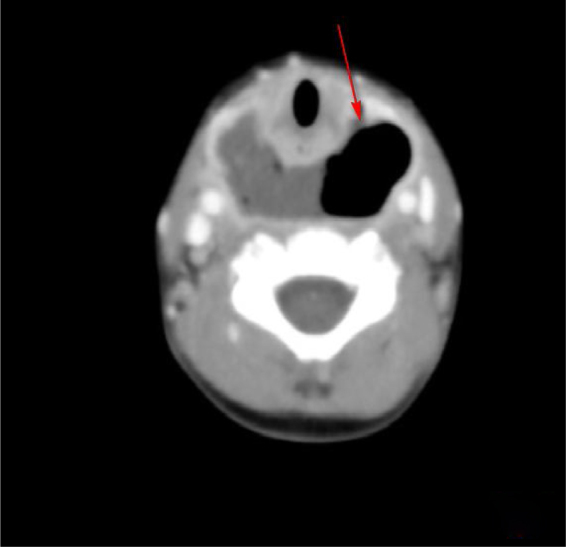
Enhanced CT: Post-pharyngeal abscess and air shadow (Horizontal scan).
